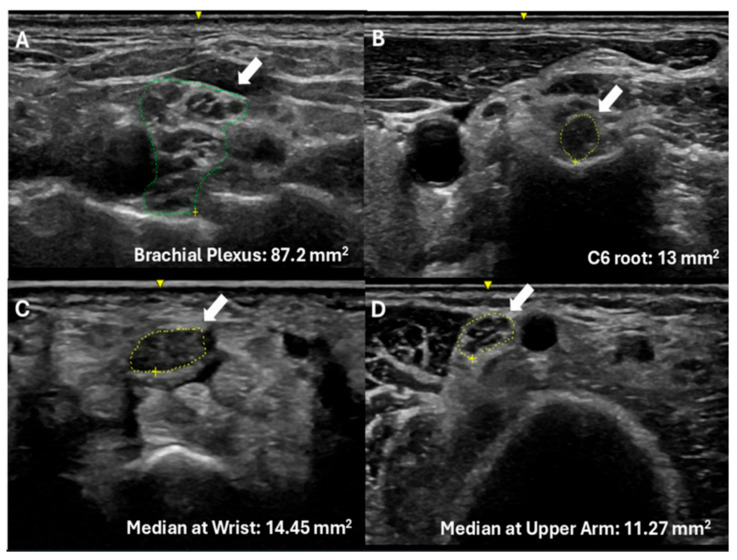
Nerve ultrasound findings in hereditary transthyretin amyloid polyneuropathy. Ultrasound images demonstrating nerve enlargement in patients with PN-ATTRv. White arrows indicate the nerve structures, while green (A) and yellow (B–D) outlines delineate their boundaries. Nerve cross-sectional areas are in mm^2^ and are provided for each panel: (A) Brachial plexus at the suprascapular fossa, (B) C6 root at the neck, (C) Median nerve at the wrist, (D) Median nerve at mid-arm.
